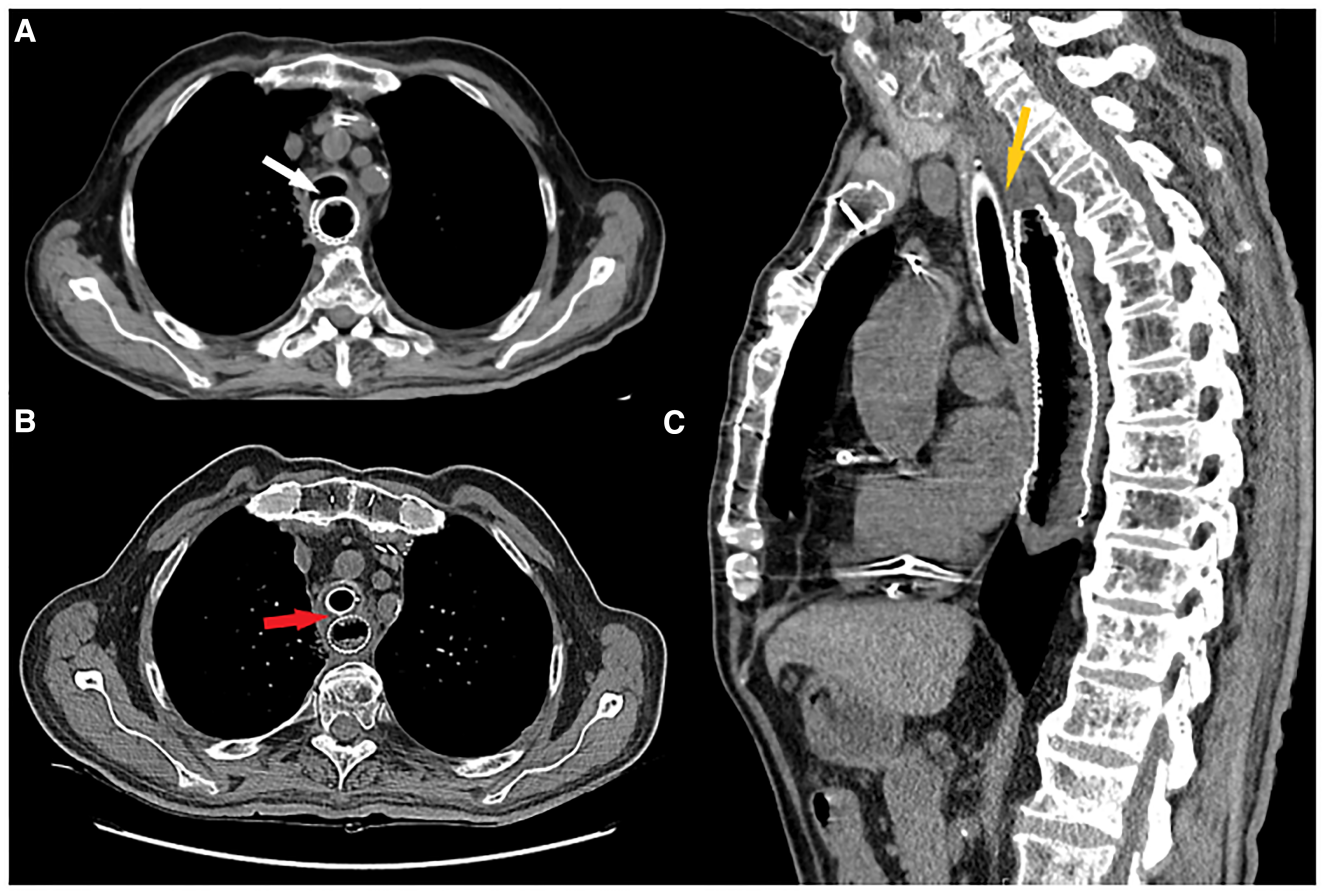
(A) Preoperative axial CT scan showing esophageal stent protruding inside tracheal lumen (white arrow). (B) Postoperative axial CT scan showing both esophageal and tracheal stent with fascia lata graft between them (red arrow). (C) Postoperative sagittal CT scan showing fascia lata graft (low density tissue between the two stents) interposed between the two stents covering the whole length of TEF.
